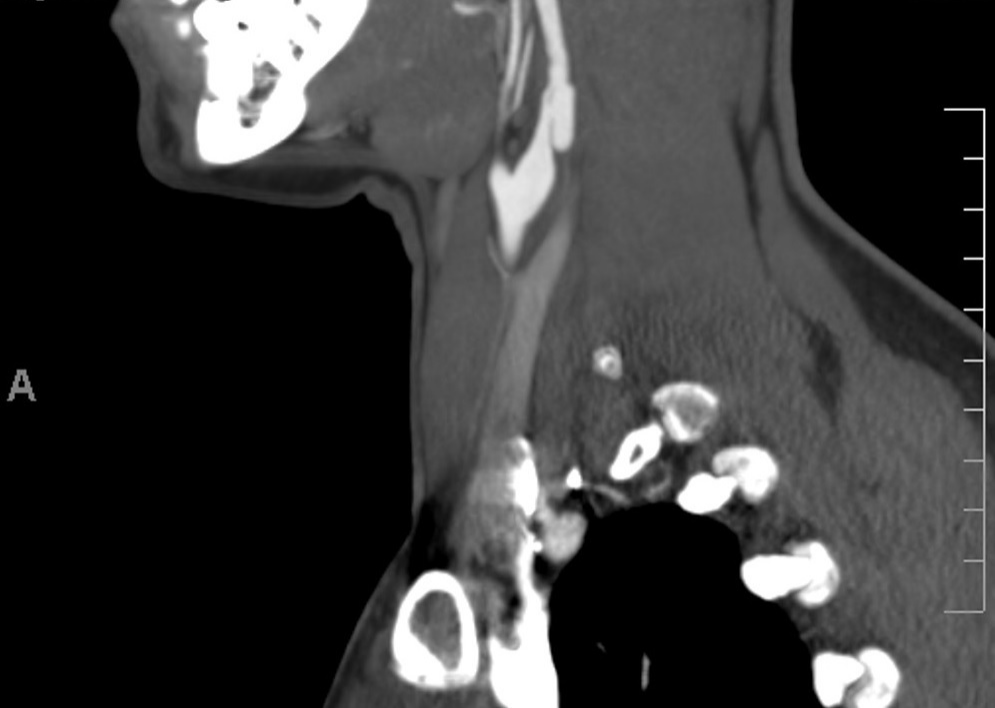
A CT angiogram showing carotid dissection
